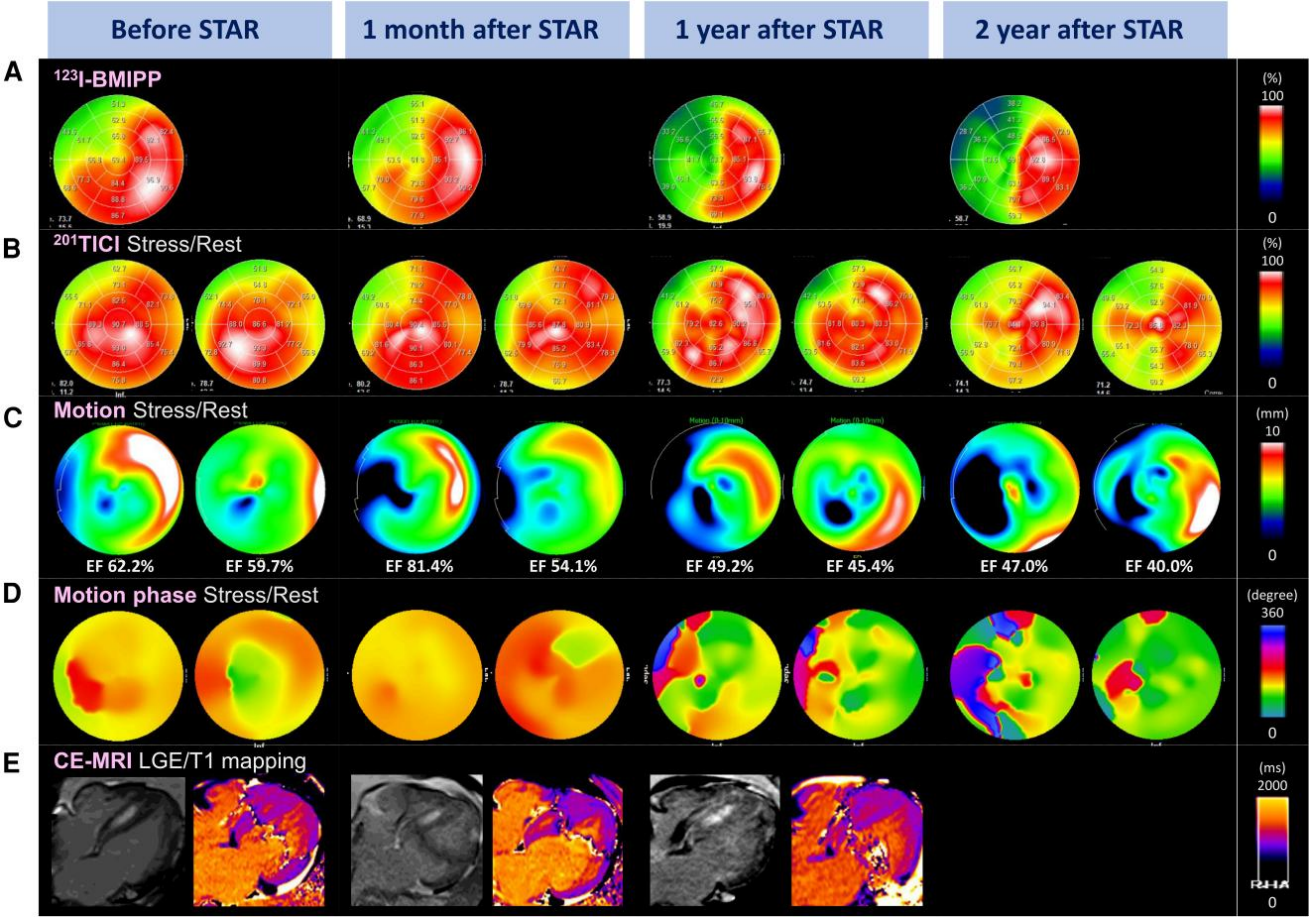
Serial imaging changes. (*A*) ^123^I-BMIPP uptake. (*B*) ^201^TlCl uptake (right, stress; left, rest). (*C*) Left ventricular wall motion (right, stress; left, rest). (*D*) Phase analysis of left ventricular motion (right, stress; left, rest). (*E*) MRI (left: LGE; right: T1 mapping). CE-MRI, contrast-enhanced cardiac magnetic resonance imaging; EF, ejection fraction; ^123^I-BMIPP, iodine-123-beta-methyl-p-iodophenyl-pentadecanoic acid; LGE, late gadolinium enhancement; STAR, stereotactic arrhythmia radioablation; ^201^TlCl, thallium-201 chloride.
